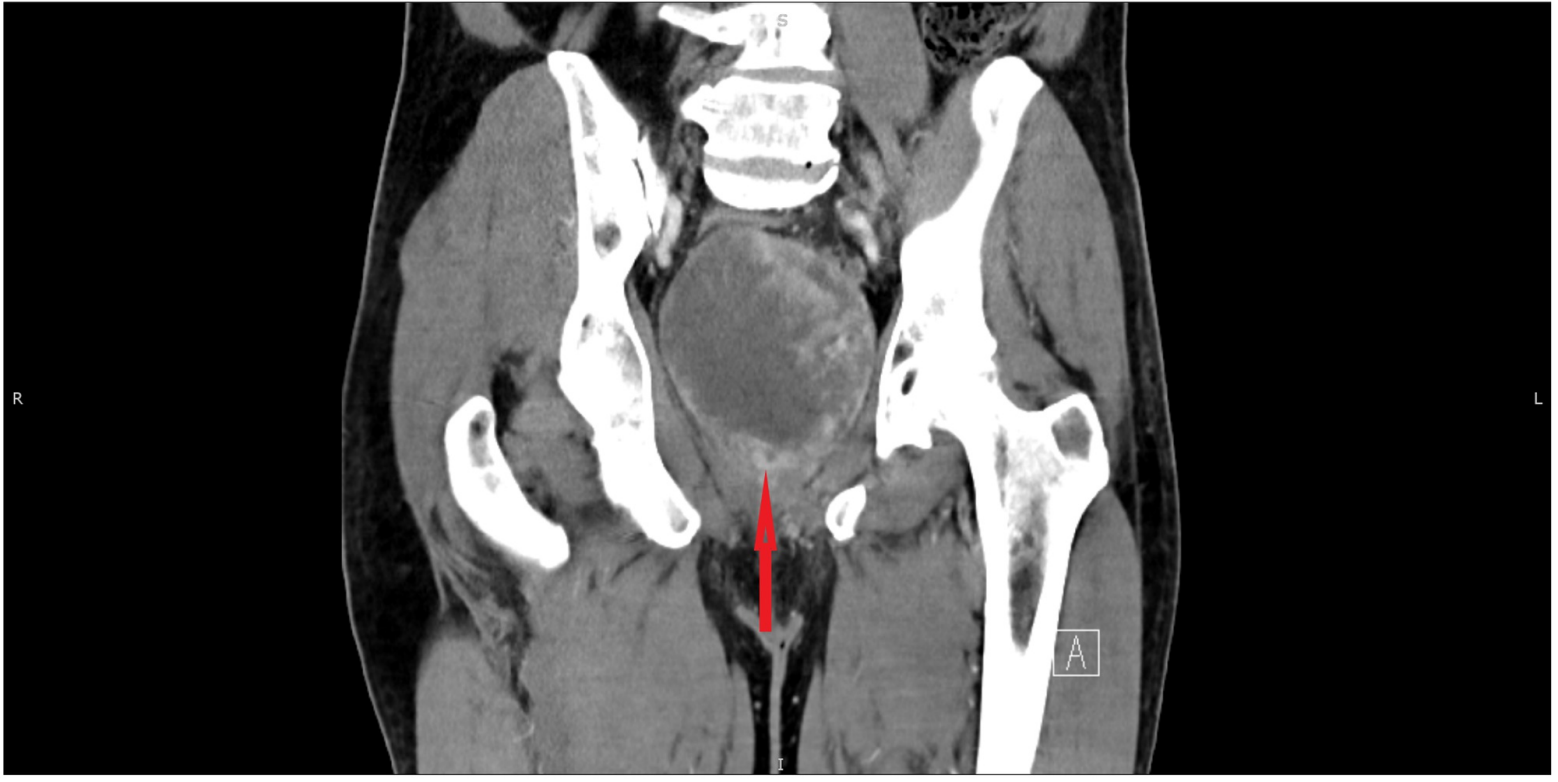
CT (coronal view) of the abdomen and pelvis with contrast showing large pelvic mass (red arrow)
